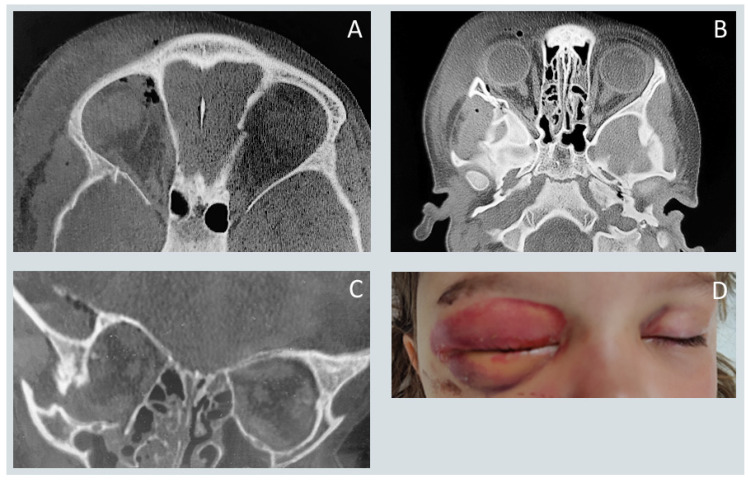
CT imaging (axial planes (A,B), and coronal plane (C)) reveals displacement of the zygomaticomaxillary complex consistent with a high-energy impact injury. Image (D) shows a 3.5-year-old child with marked periorbital edema and ecchymosis following a road traffic accident.
